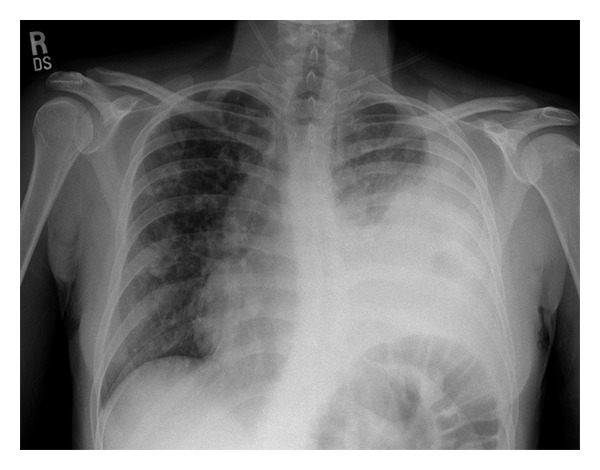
Chest radiograph showing left-sided pleural effusion shortly after admission.
